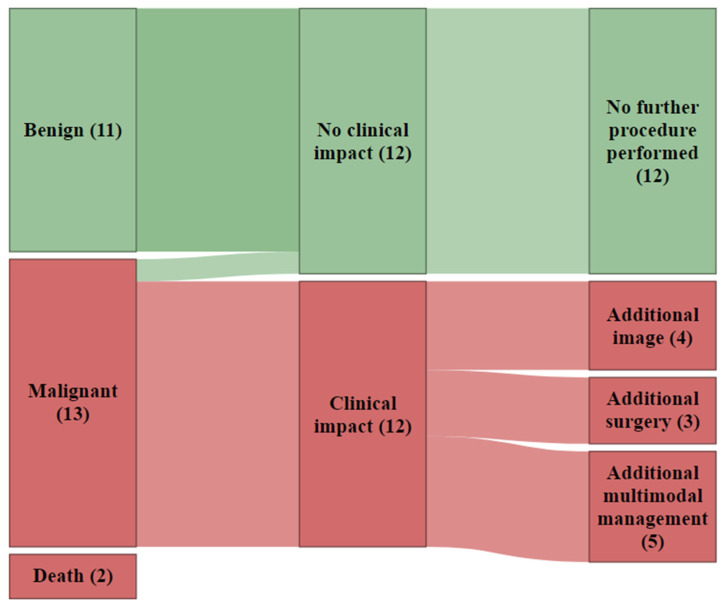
Sankey plots displays, whether patient management was influenced by findings reported as suspicious of additional malignancy on FDG-PET/CT at staging in NSCLC patients with regards to their nature after further examinations following FDG-PET/CT.
